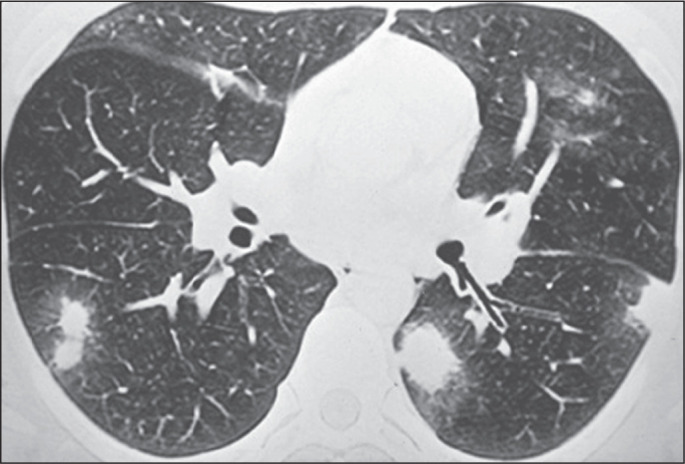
CT of a 47-year-old woman, showing multiple nodules of varying sizes
